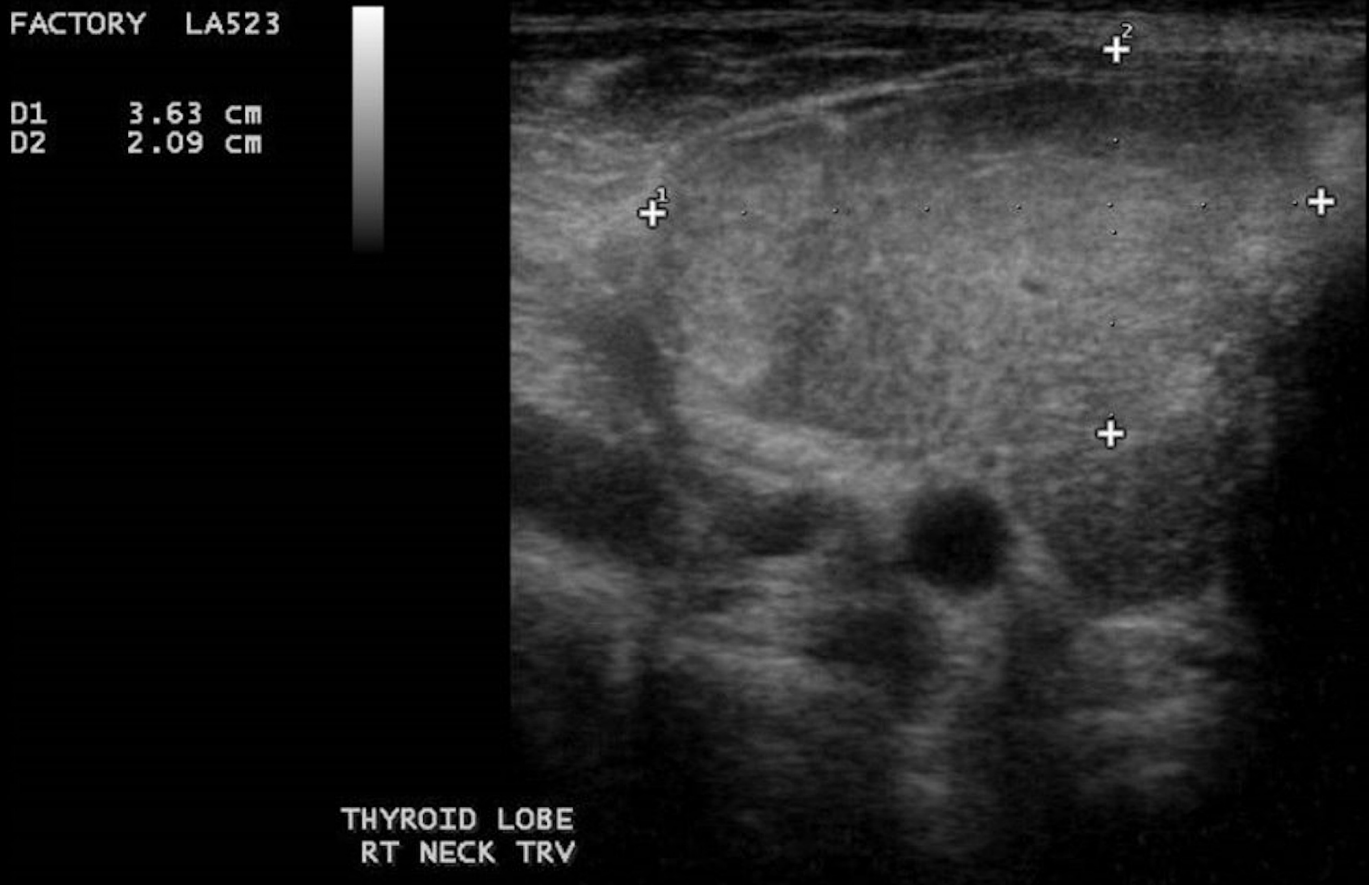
Ultrasound of the right thyroid lobe
